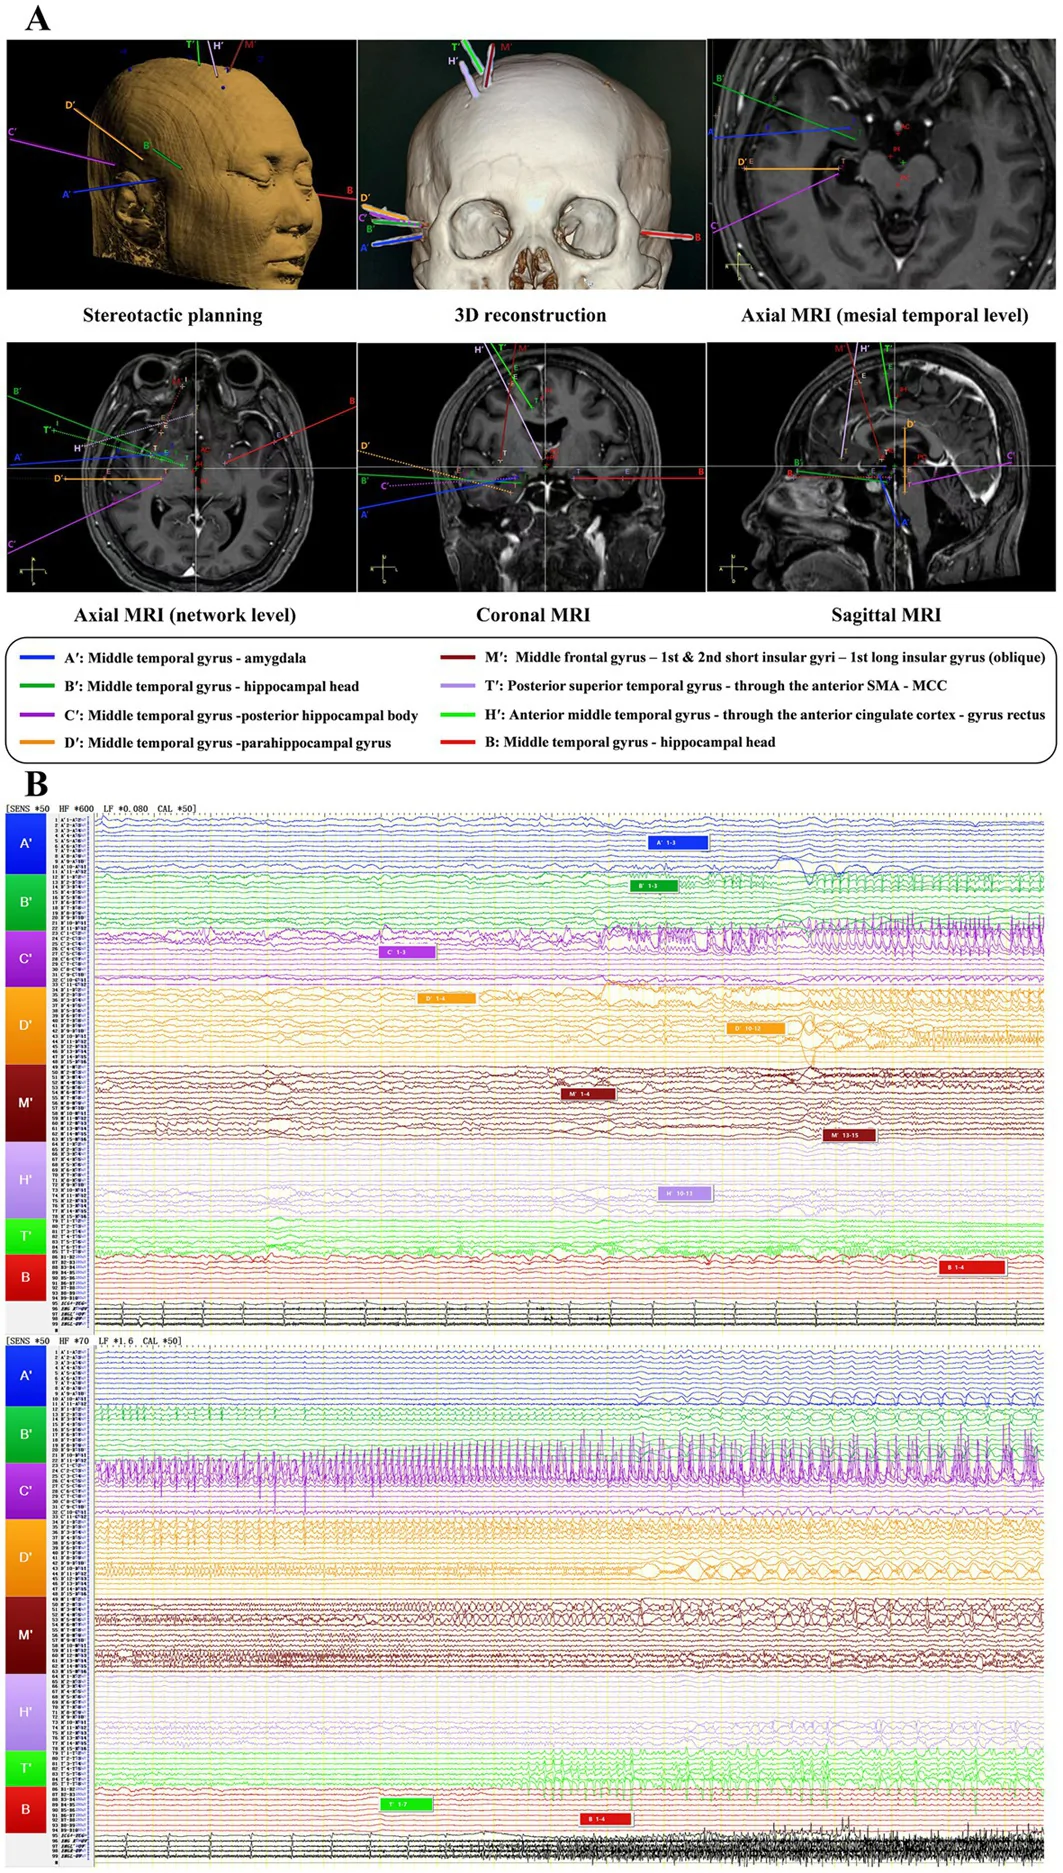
SEEG electrode implantation and representative ictal recordings. (A) Three-dimensional reconstruction and multiplanar MRI showing the implantation configuration and intracranial trajectories of the SEEG electrodes. (B) Postoperative SEEG recordings demonstrated that the right hippocampus and parahippocampal gyrus were the seizure onset zones. The onset of abnormal epileptiform discharges recorded by the right deep electrodes corresponded to the lesion identified on MRI.
